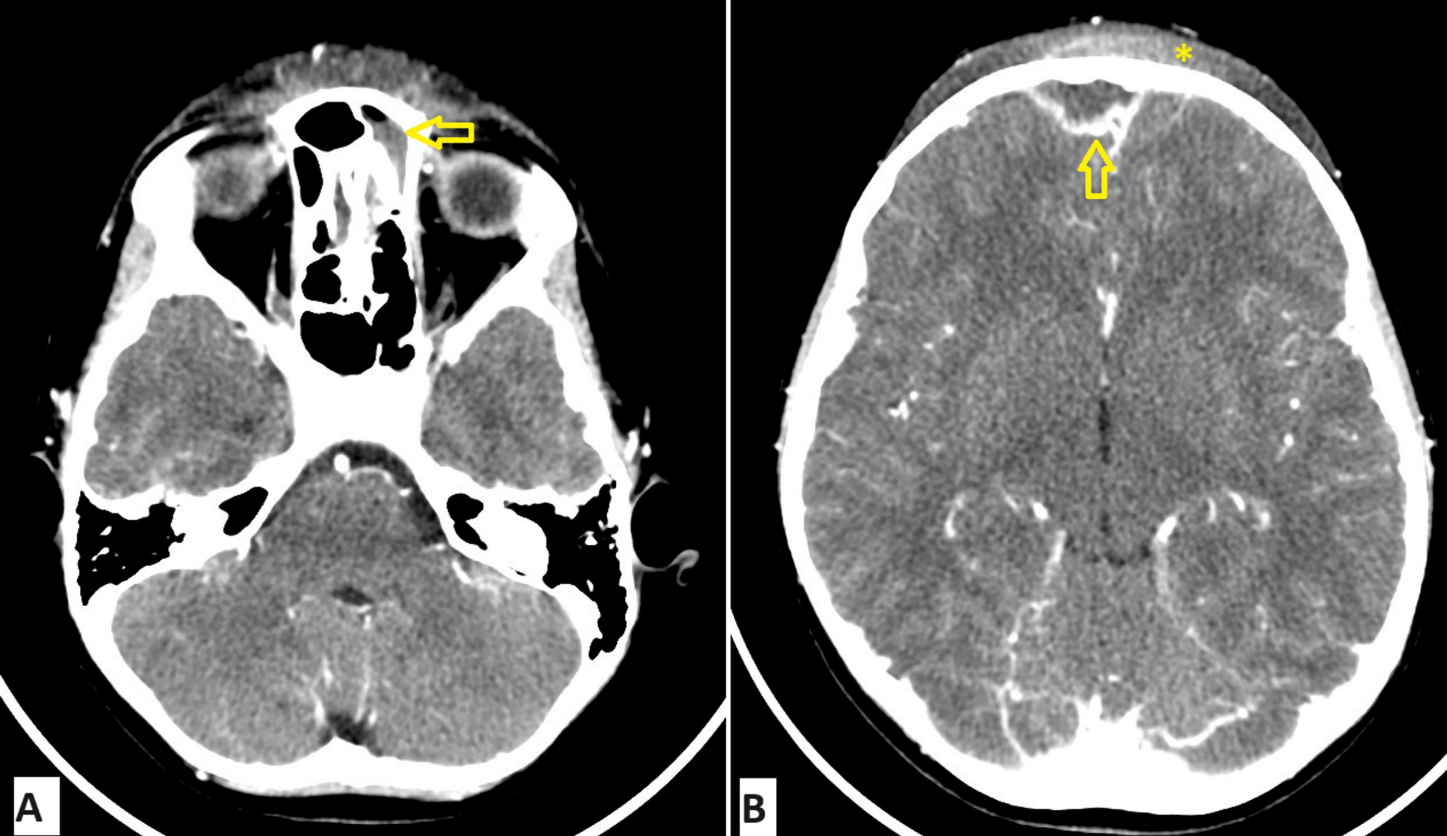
CT brain with contrast done upon presentation. Panel (A) demonstrates soft tissue swelling and frontal sinus mucosal thickening more on the left with a small air-fluid in the left frontal sinus. Panel (B) shows subgaleal and subperiosteal fluid collections and an extra-axial collection at the right frontal convexity with a peripheral rim of enhancement. Horizontal arrow: left frontal sinusitis with air-fluid level; vertical arrow: extra-axial collection measuring 1.2 cm in thickness extending over 3 cm; asterisk: scalp swelling with subgaleal and subperiosteal fluid collections
